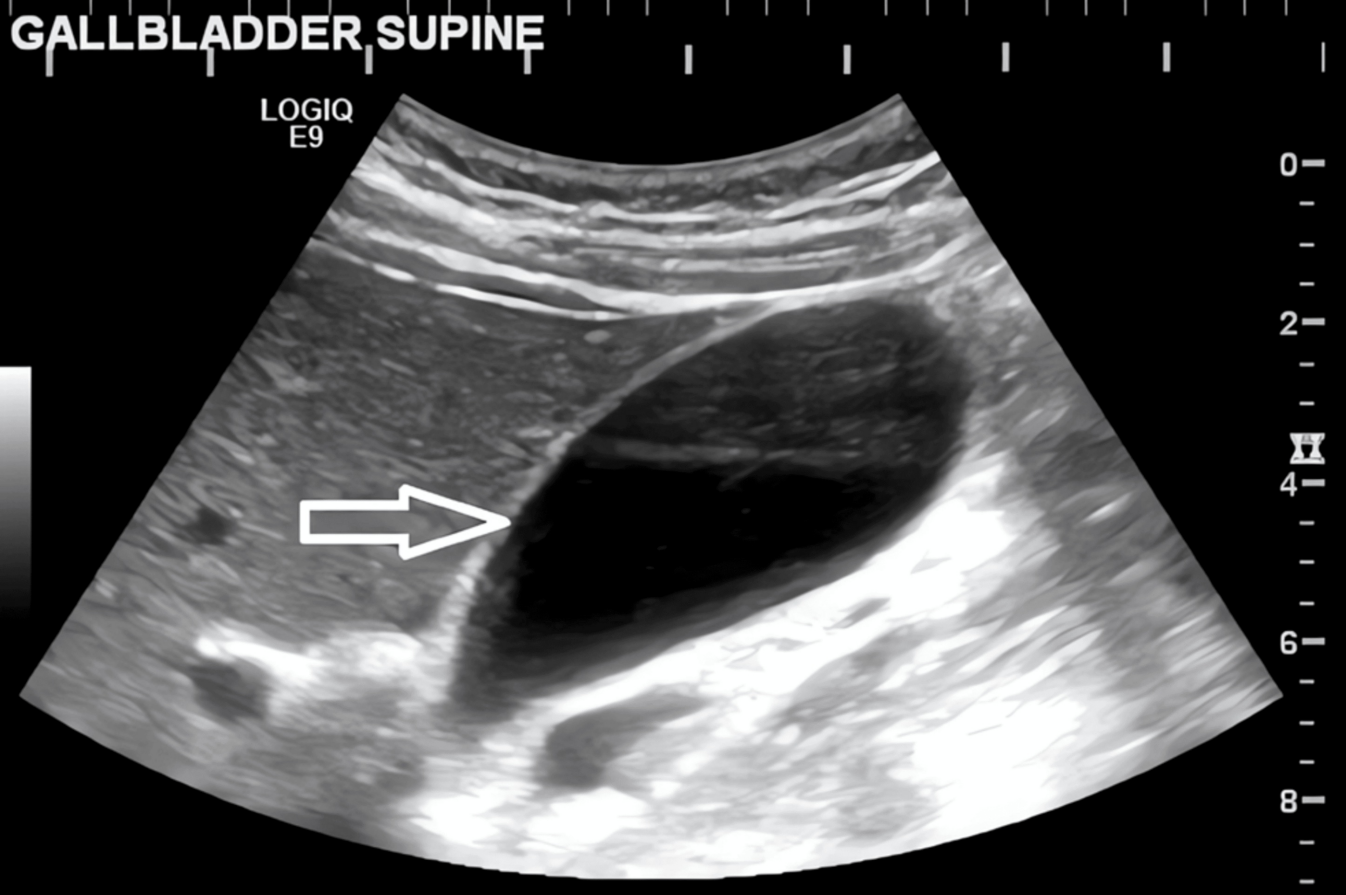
Ultrasound image of the gallbladder showing minimal biliary sludge This ultrasound scan (longitudinal view) demonstrates mildly echogenic material (white arrow) within the gallbladder lumen, consistent with biliary sludge.
